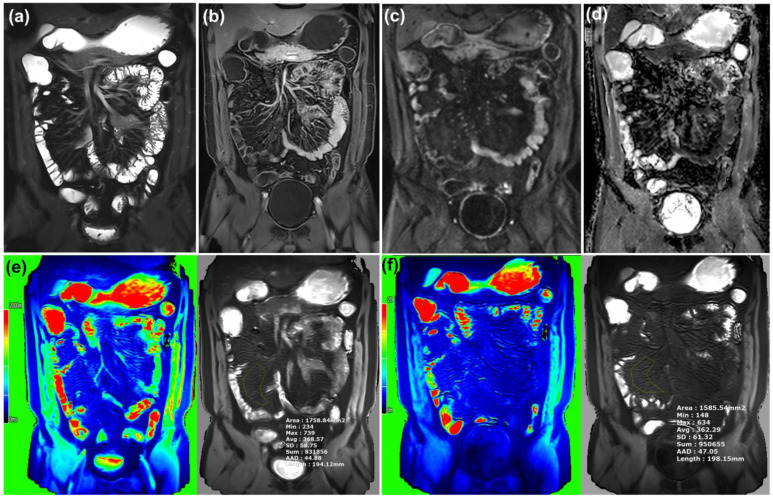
Images from a patient with inactive Crohn’s disease (CDAI = 31.1). No significant imaging sign of active bowel inflammation on T2WI (a), postcontrast T1WI (b), DWI b-900 (c), and ADC (d). The coronal native T1 map (e) demonstrates a T1 relaxation time of 368.6 ms. Post-enhanced T1 map (f) demonstrates a T1 relaxation time of 362.3 ms. ΔT1 was 6.3 ms.
